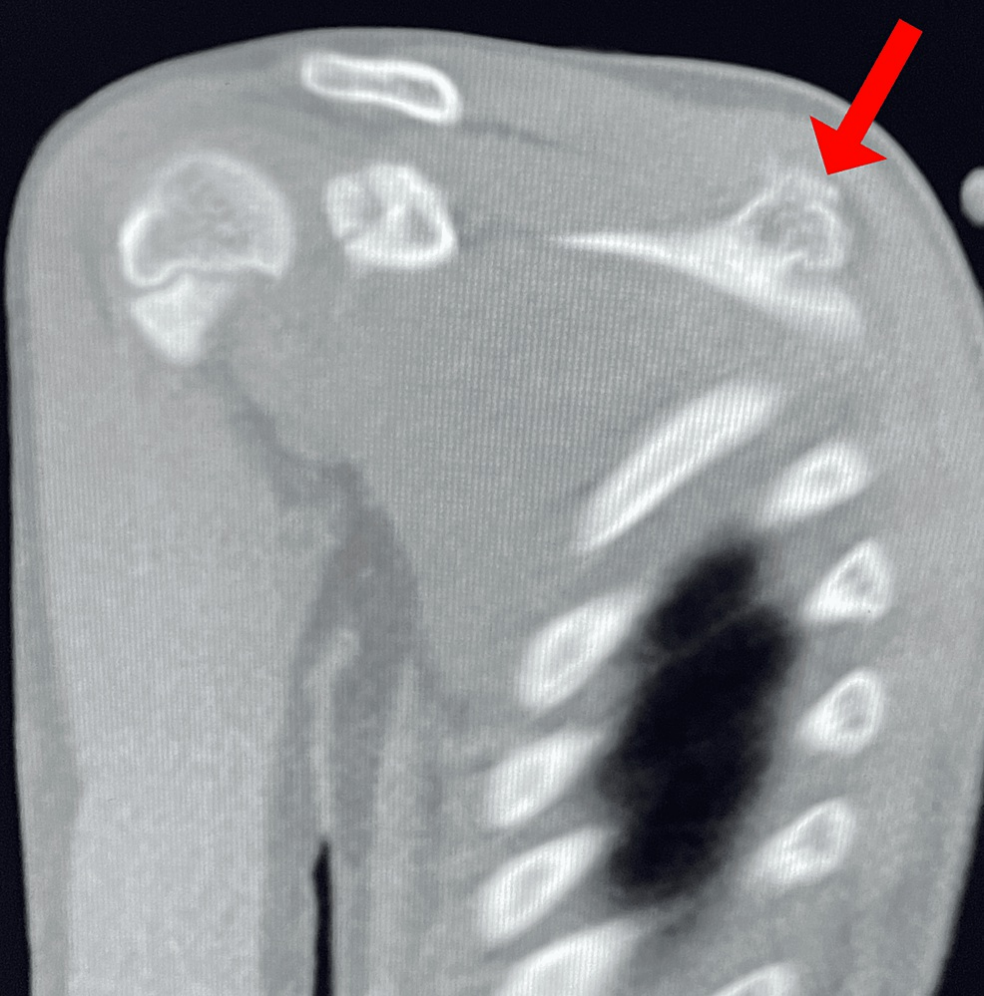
CT scan showing abnormal bone growth. The image shows an exostosis of the right scapula (arrow).
